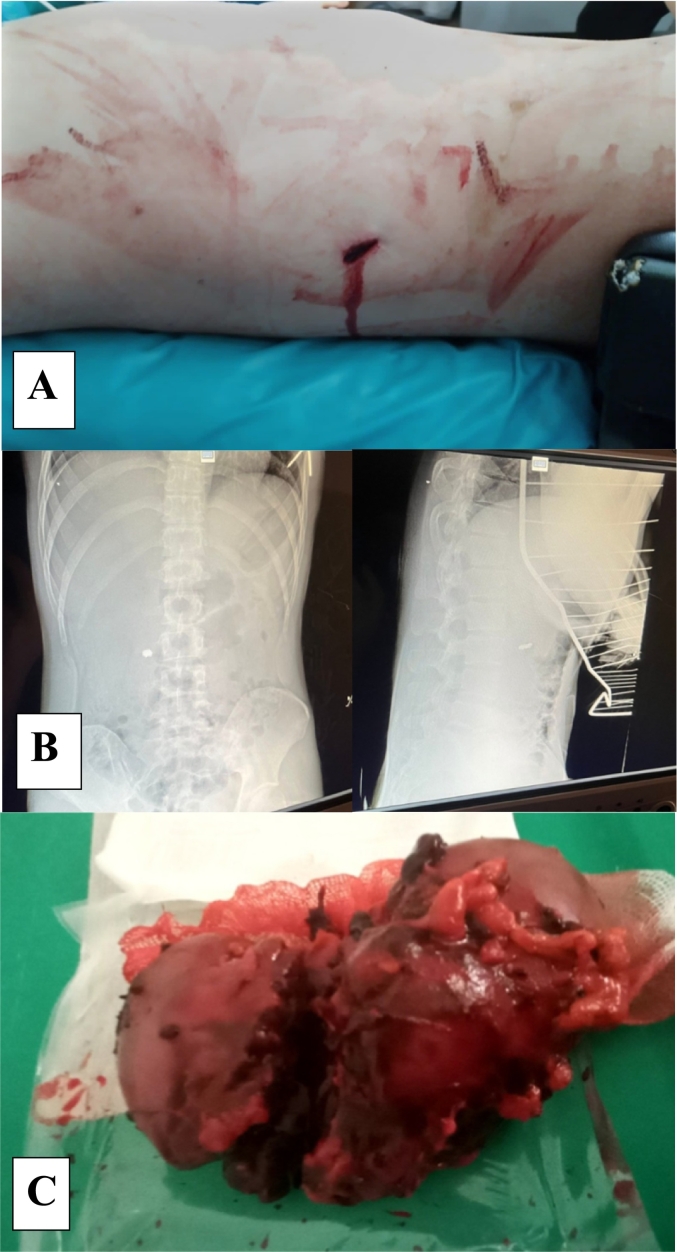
Wounded B., (A - The entrance to fire hole of the right lumbar area; B - X-ray in the direct and lateral projection of the organs of the abdominal cavity - presence of a foreign body - a metal fragment in the right side of the abdomen; C - macropreparation, right kidney removed after its damage by gunshot fragment - V degree according to the AAST classification.)
